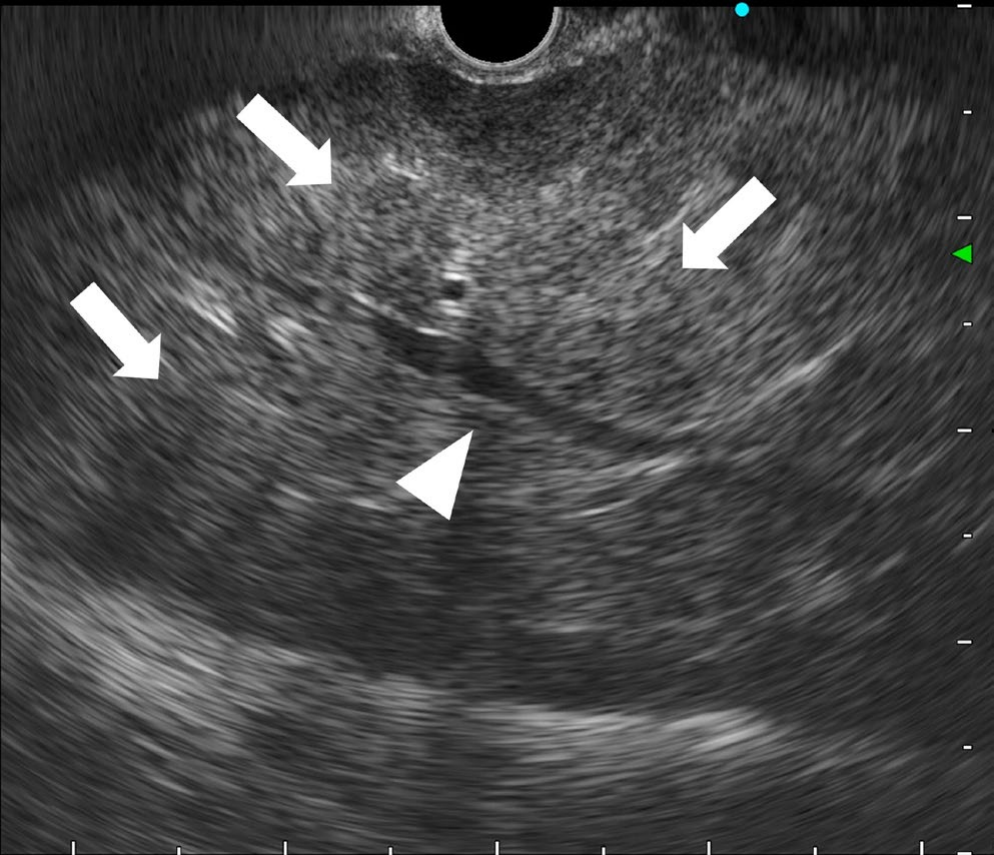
Endoscopic ultrasonography demonstrates multiple ill‐defined, hypoechoic hepatic masses diffusely distributed in the left hepatic lobe (arrow). The lesions show relatively homogeneous internal echoes without a distinct capsule, with preserved intrahepatic vascular structures (arrowhead), reflecting a compressive growth pattern characteristic of malignant lymphoma. A potential pitfall is a false‐negative result due to inadvertent sampling of normal hepatic parenchyma. Therefore, even in ill‐defined lesions, careful EUS observation is essential, and targeted biopsy is performed toward hypoechoic areas that are confidently considered to represent the lesion.
